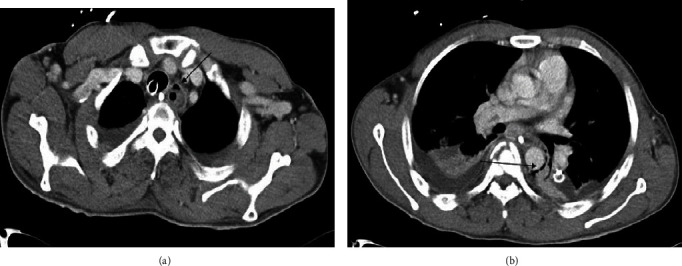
Chest CT scan shows (a) air leak around the esophagus and (b) pneumomediastinum (arrows).
